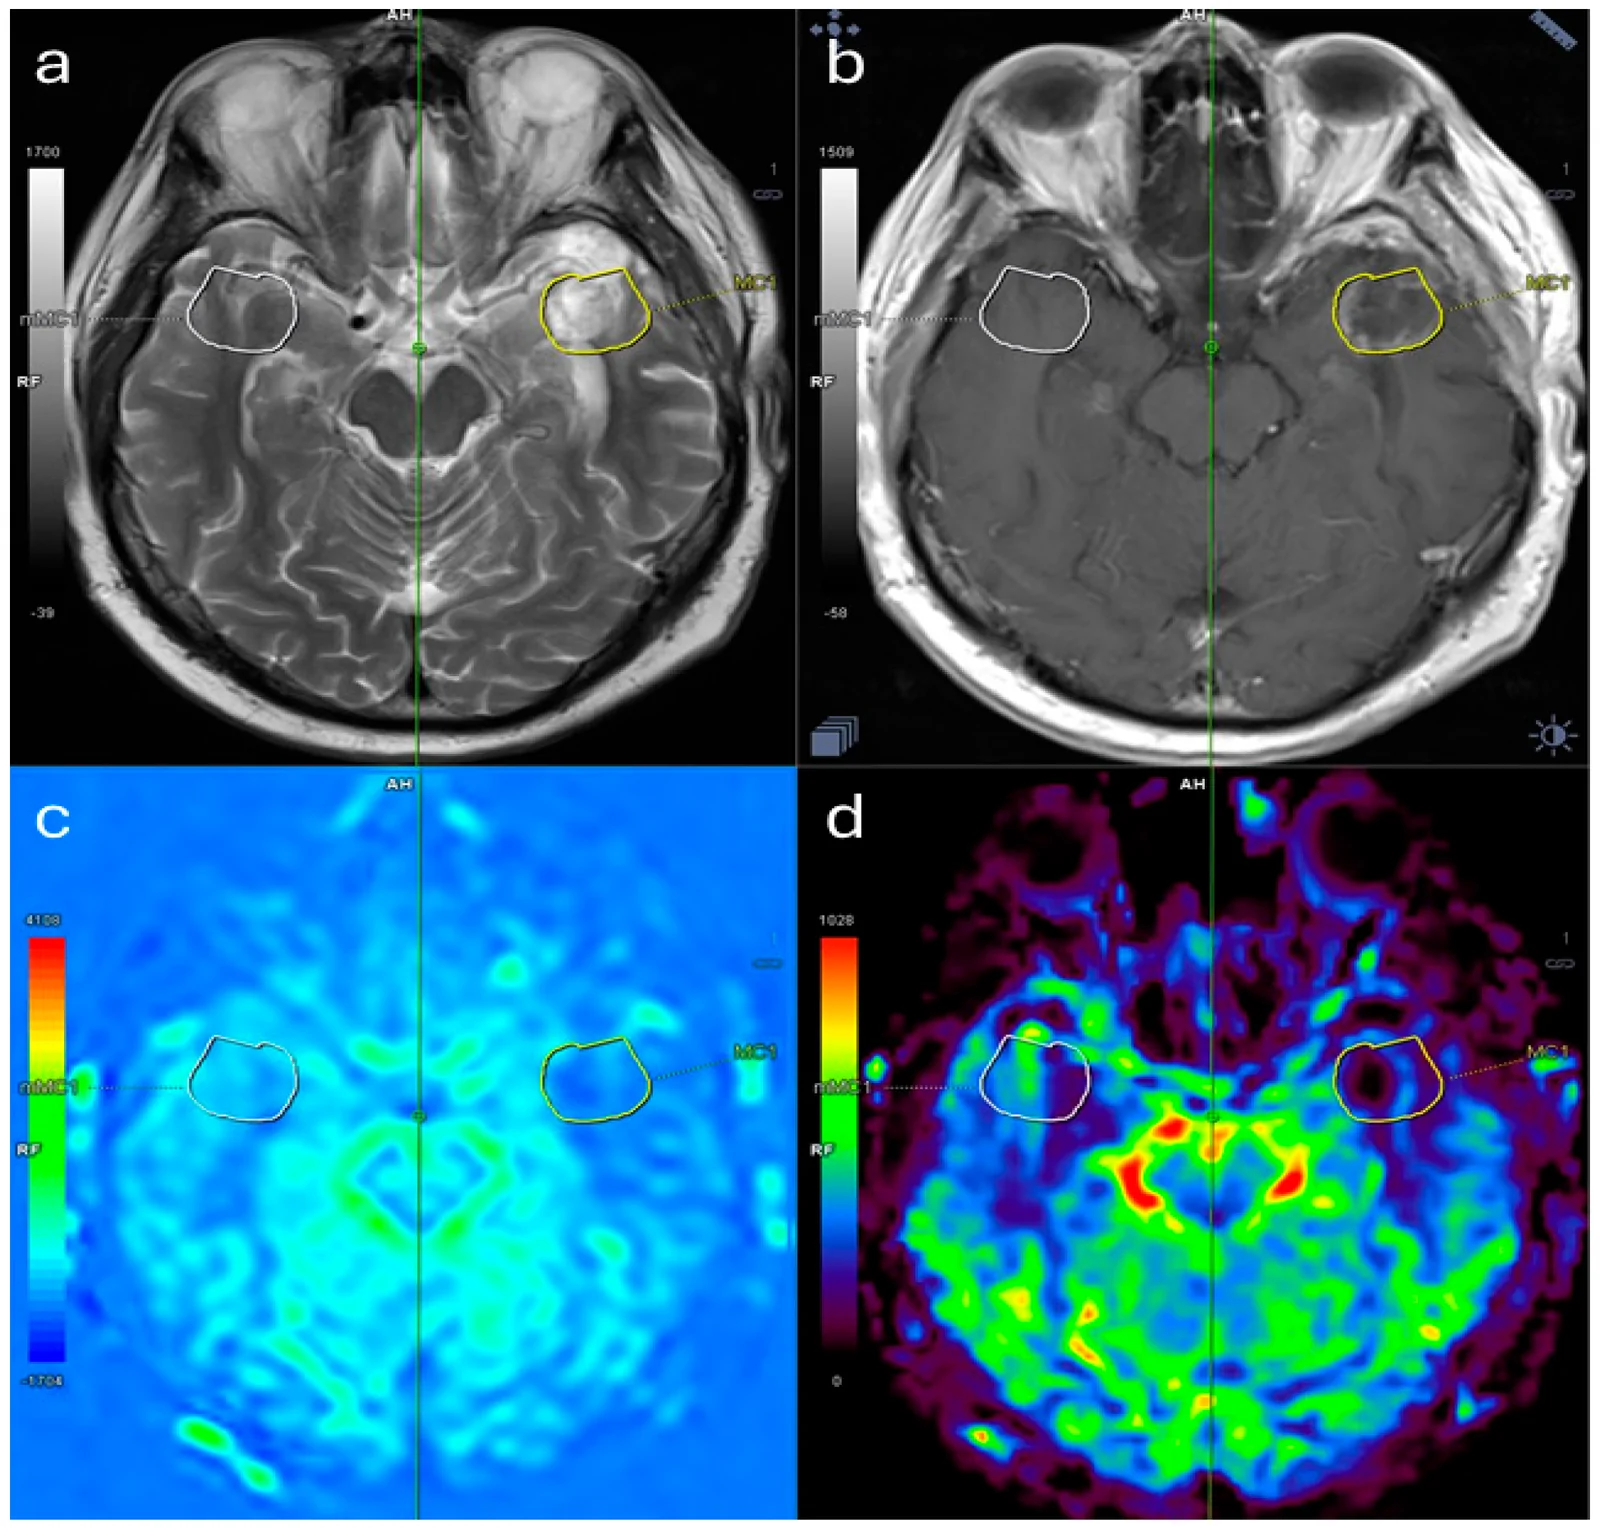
Treatment-related changes in a 58-year-old male patient with a history of glioblastoma. Axial T2-weighted (a) and post-contrast T1-weighted (b) images show postoperative changes without a mass-like lesion. Arterial spin labeling (ASL) (c) and dynamic susceptibility contrast (DSC) (d) perfusion images show no focal hyperperfusion. Colored outlines indicate the lesion region of interest, and white outlines indicate the corresponding contralateral reference region used for comparison.
